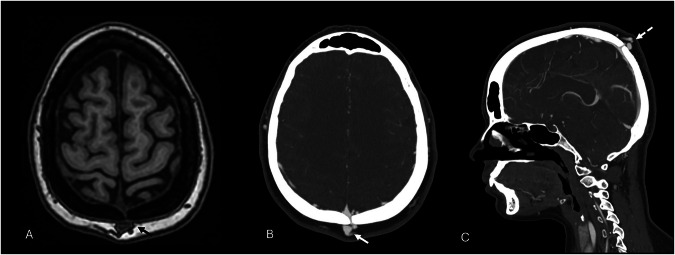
Axial 3D T1-W image shows some anomalous dilated vessels (black arrow) within scalp tissues (A). Axial and sagittal images from a CT with venous phase, demonstrate the anomalous connection between the subgaleal vein and the superior sagittal sinus (white continuous and dotted arrows) within a defect of the parietal bone (B, C)
